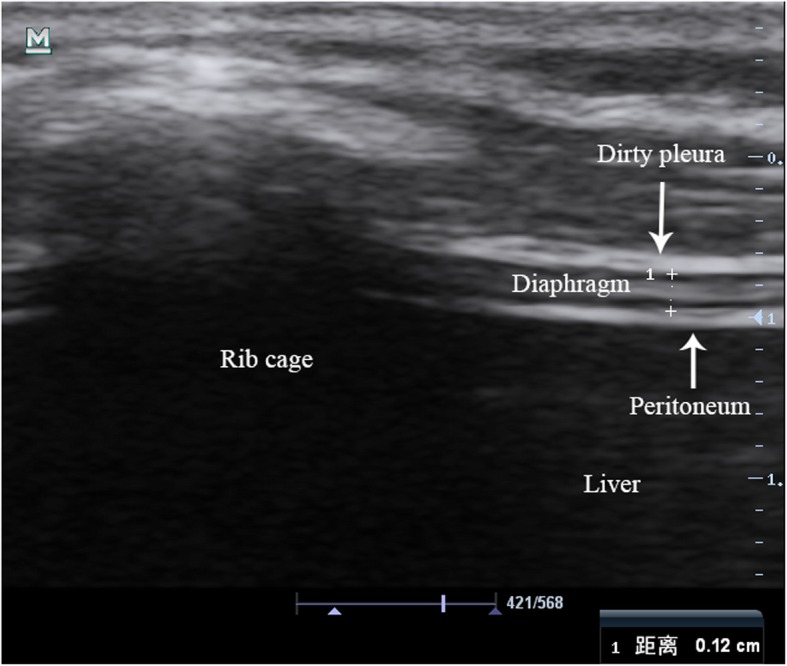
Ultrasound B-mode using a 10 MHz probe in the zone of apposition
